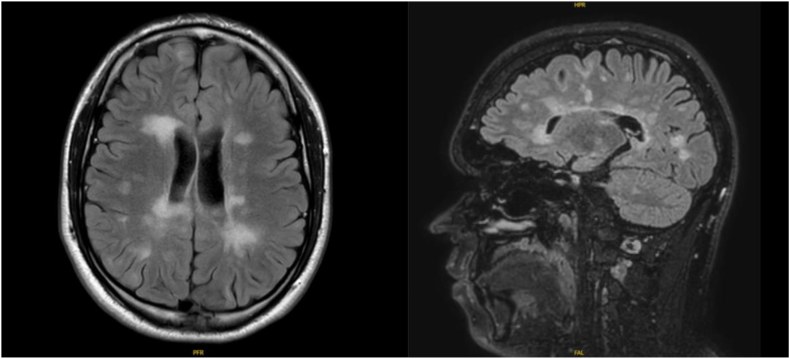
Axial and Sagittal FLAIR MRI scans of the brain demonstrating high lesion load at presentation in a 14 year-old child with MS. Note the presence of multiple discrete hyperintense lesions involving the supratentorial white matter and posterior fossa, involving the cerebellar peduncles and the cerebellar hemispheres bilaterally.
